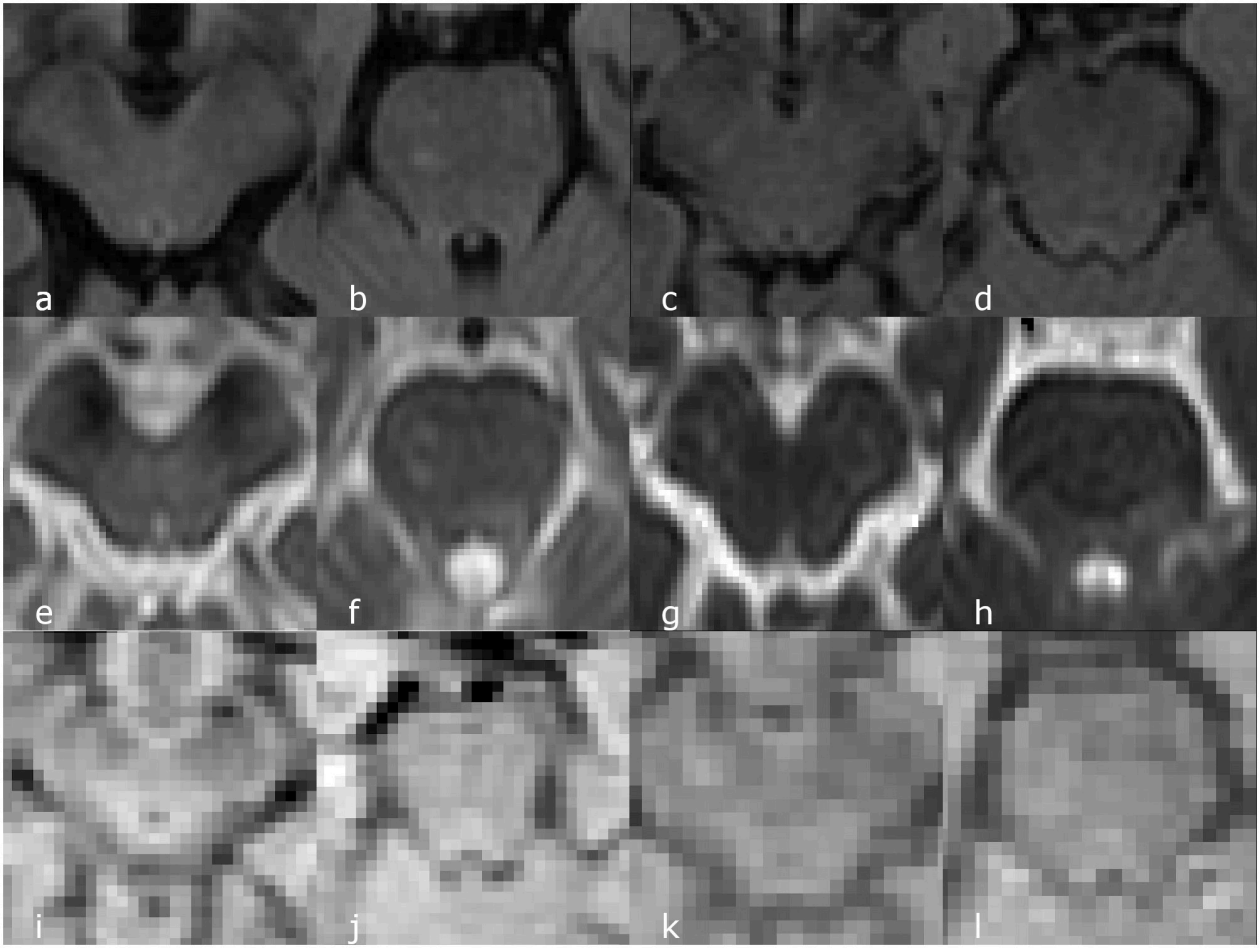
Example images. Example FLAIR images from a iNPH patient (a, b) and control (c, d) with corresponding ADC (e-h) and time averaged perfusion raw data (i-l) at the level of the mesencephalon and pons.
